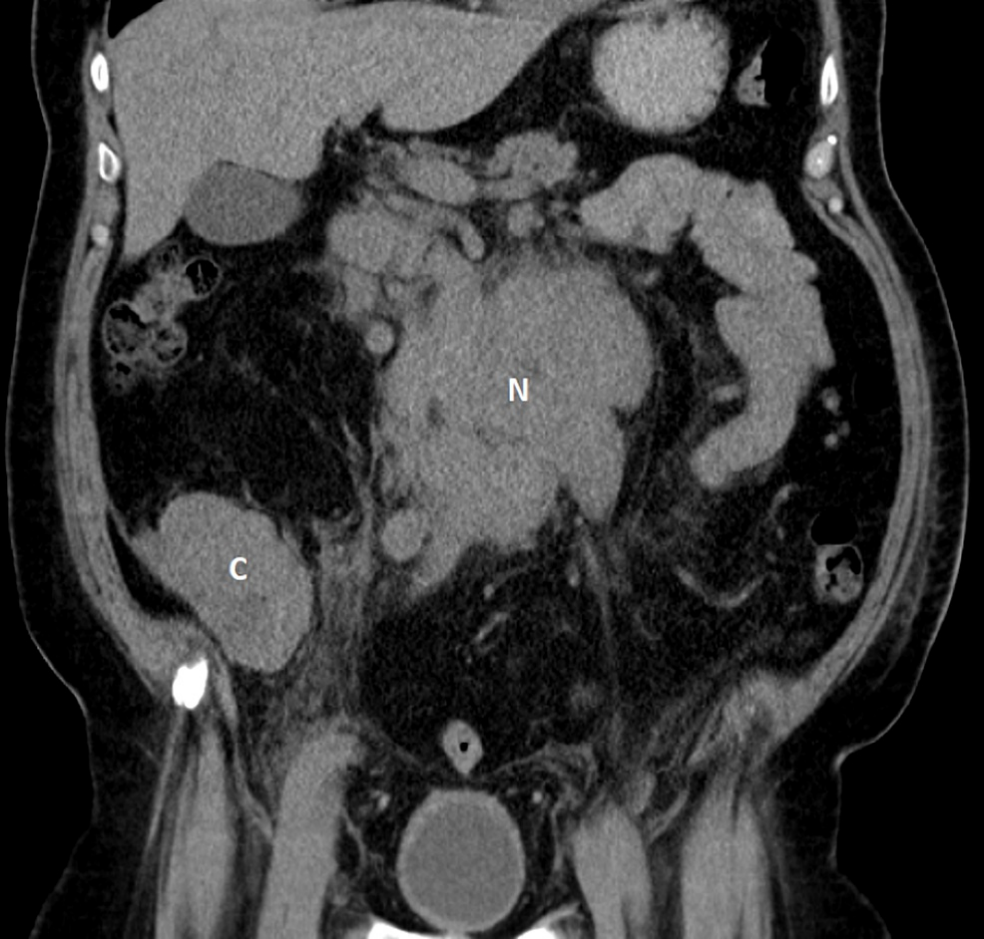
Computed tomography of the abdomen (coronal plane) showing a severe cecal wall thickening (C) and a conglomerate lymph nodal mass (N)
